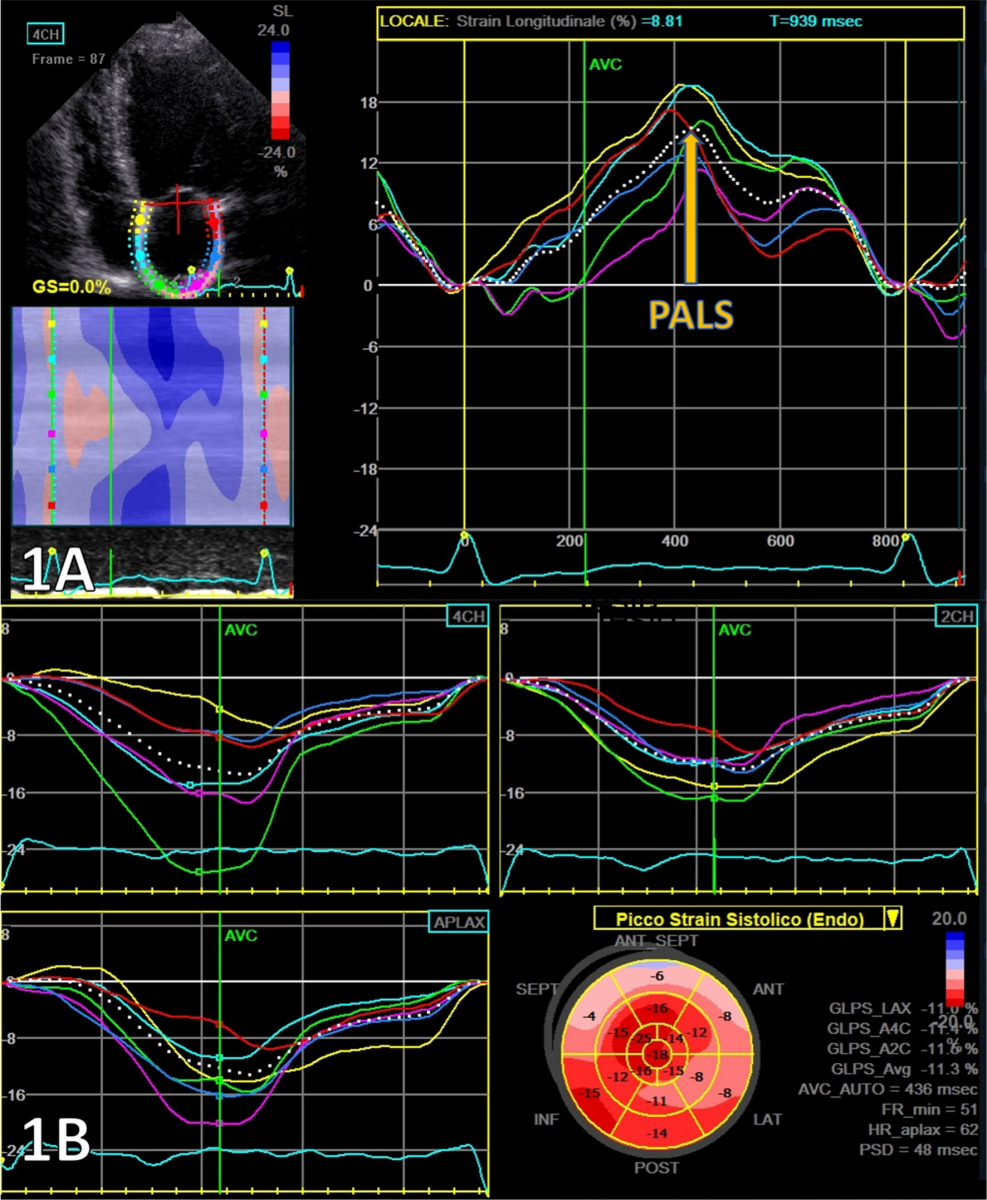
Representative example of two-dimensional STE–derived PALS measurements A and LV GLS B from an apical four-chamber view
